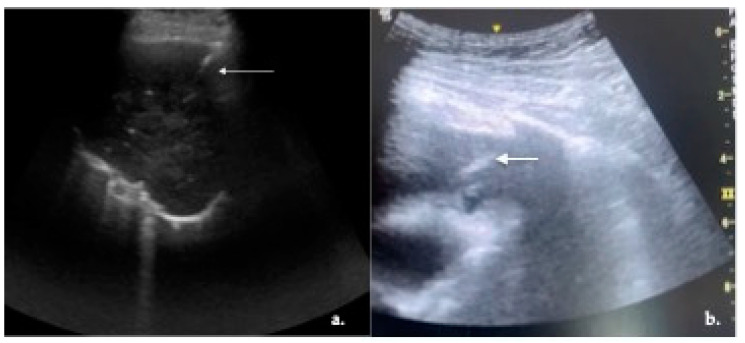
Ultrasound-guided percutaneous lung lesion biopsy (US-PLLB); (a) in-plane 18 G US-PLLB in patient with not otherwise specified non-small cell lung cancer (NOS-NSCLC); white arrow: needle; (b) in-plane 16 G US-PLLB in patients with non-Hodgkin lymphoma (NHL); white arrow: needle.
